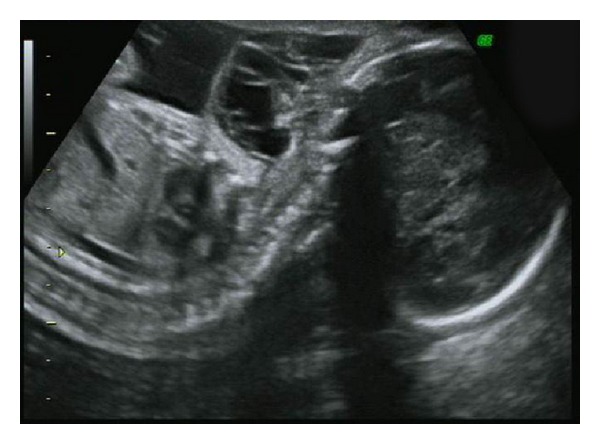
Ultrasound image at 26 weeks' gestation of a cervical teratoma.
